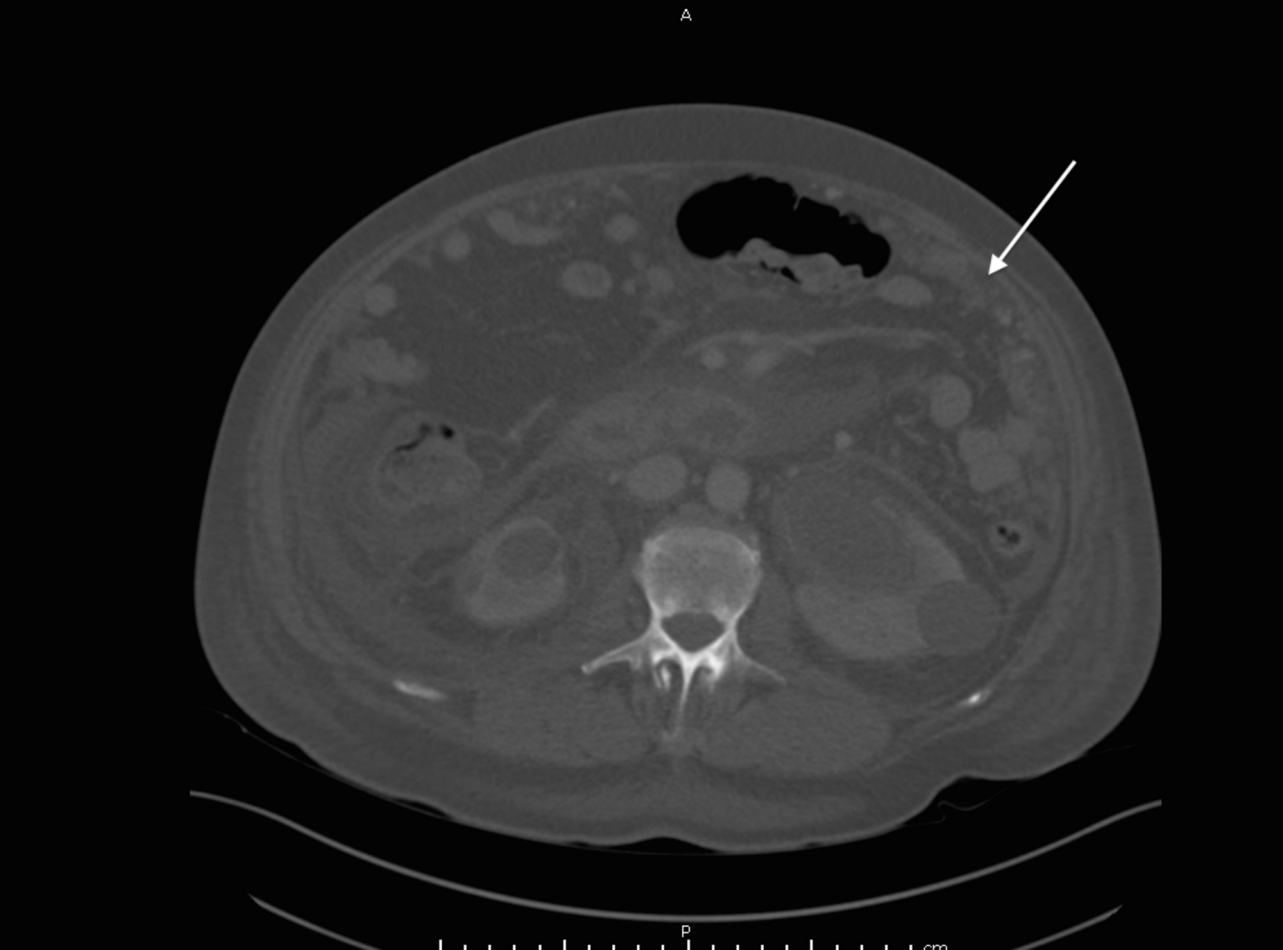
Peritoneal carcinomatosis CT abdomen and pelvis scan performed without intravenous contrast demonstrating peritoneal carcinomatosis CT: computed tomography
